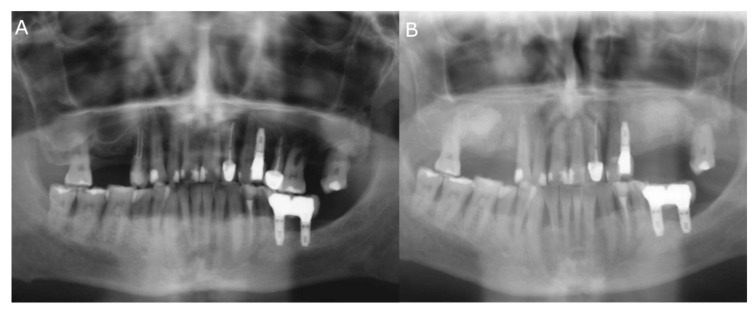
(A) Preoperative and (B) postoperative orthopantomography radiography after bilateral sinus lift using the lateral window approach technique.
